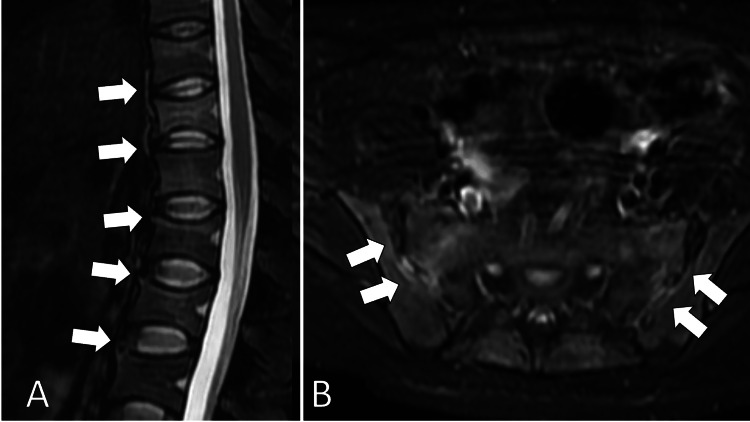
Magnetic resonance imaging of the thoracic and lumbar vertebra (A) and pelvis (B) confirming the inflammation of the vertebral and sacroiliac joints (white arrows)
